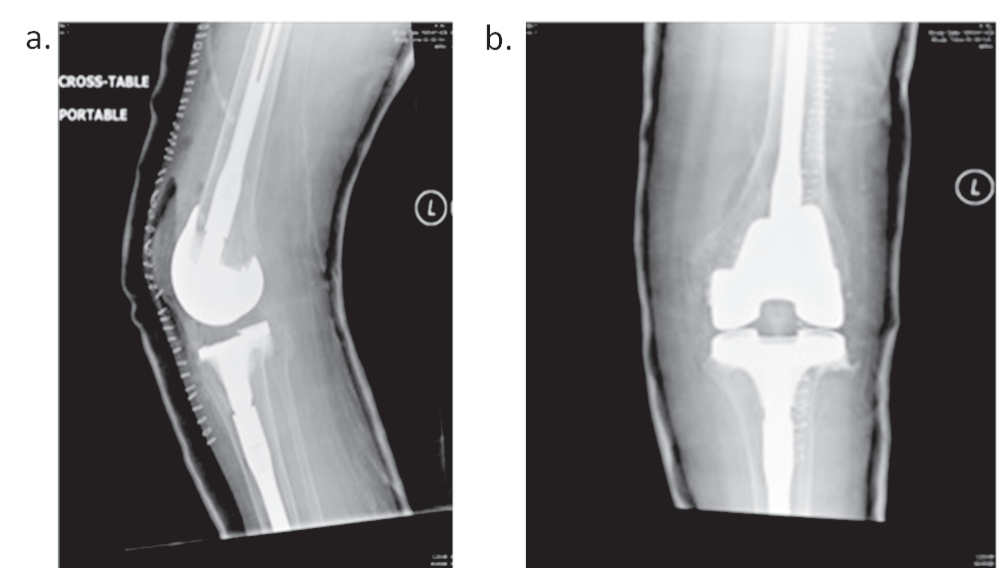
X-ray of left knee post TKR.
